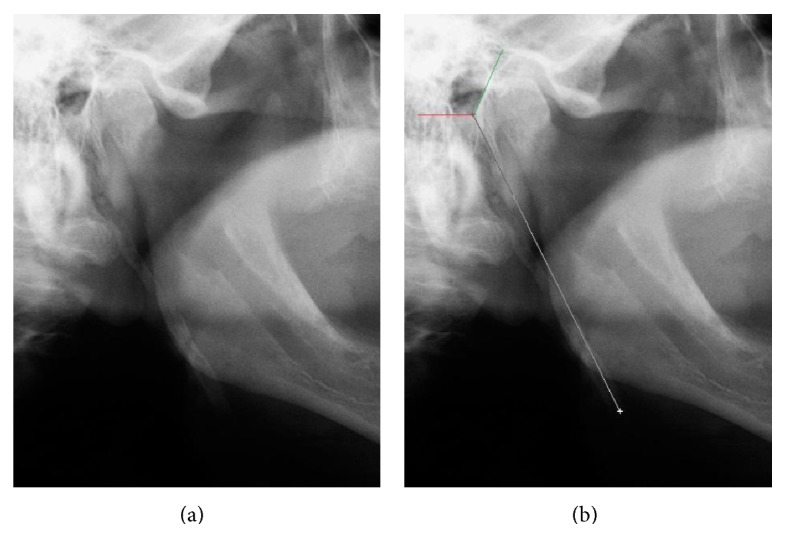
(a) Panoramic radiography showing the elongated styloid process. (b) Measurement of the styloid process using the software's rule (the red line shows the lower limit of the external ear canal and the green the previous limit).
